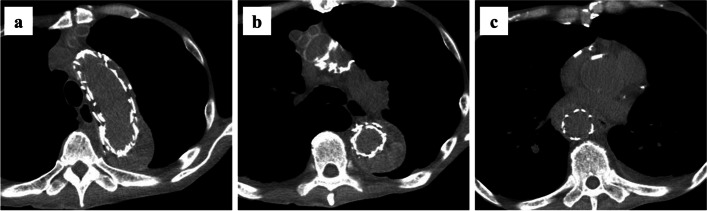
Plain computed tomography at 6 months after the total arch replacement. No obvious change in aortic diameter is observed compared with the previous examination or compression of the stent graft by the false lumen
